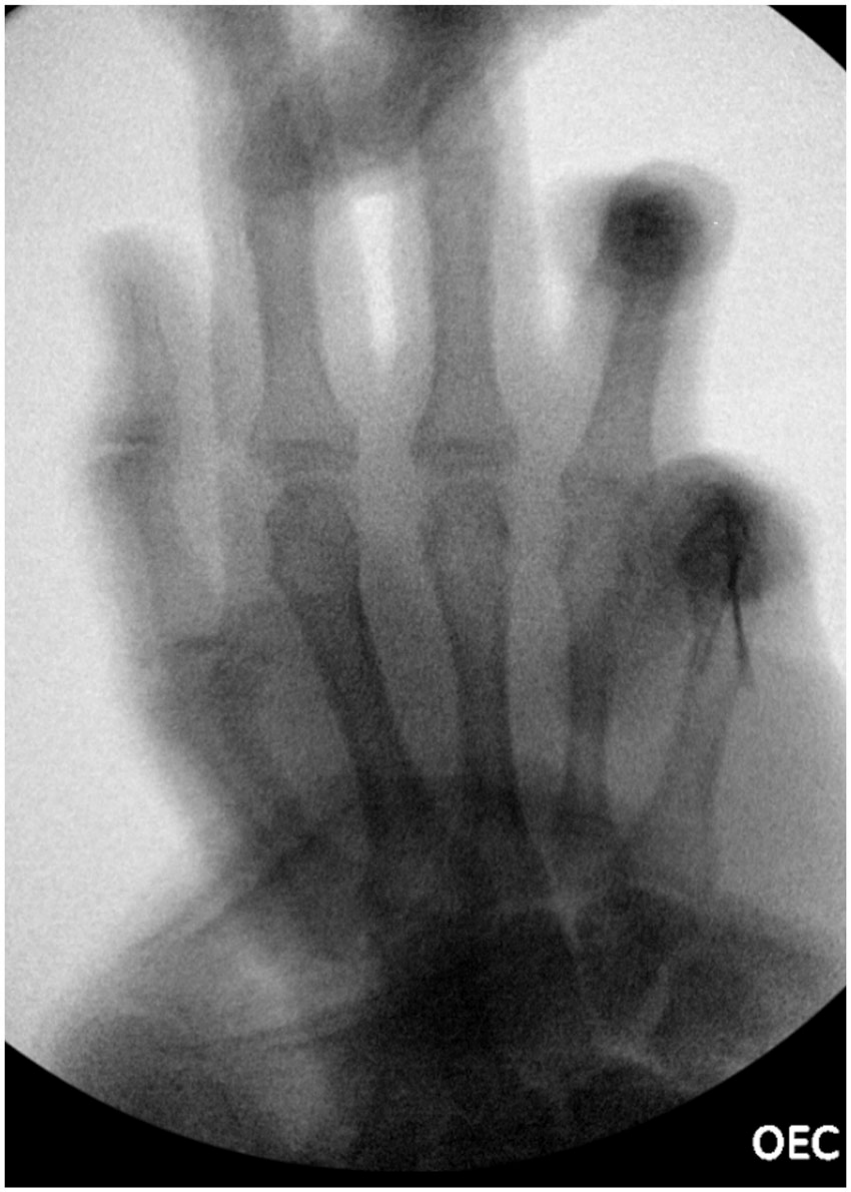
X-ray of the right-hand showing K-wire fixation.
